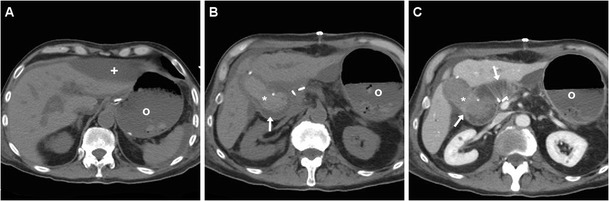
Biloma and intraluminal haemorrhage in a 72-year-old man after pylorus-preserving PD for CBD cholangiocarcinoma and initial diagnosis of postoperative PF treated conservatively. On postoperative day 16, precontrast (a) and contrast-enhanced (b, c) CT showed fluid-attenuation collection (+) located ventrally and inferiorly to the left liver lobe, consistent with bile; distended stomach (o) with fluid indicating DGE, hyperattenuating blood (*) in the distended MJL (*arrows*). Without CT evidence of active bleeding, the patient was treated conservatively with blood transfusions
